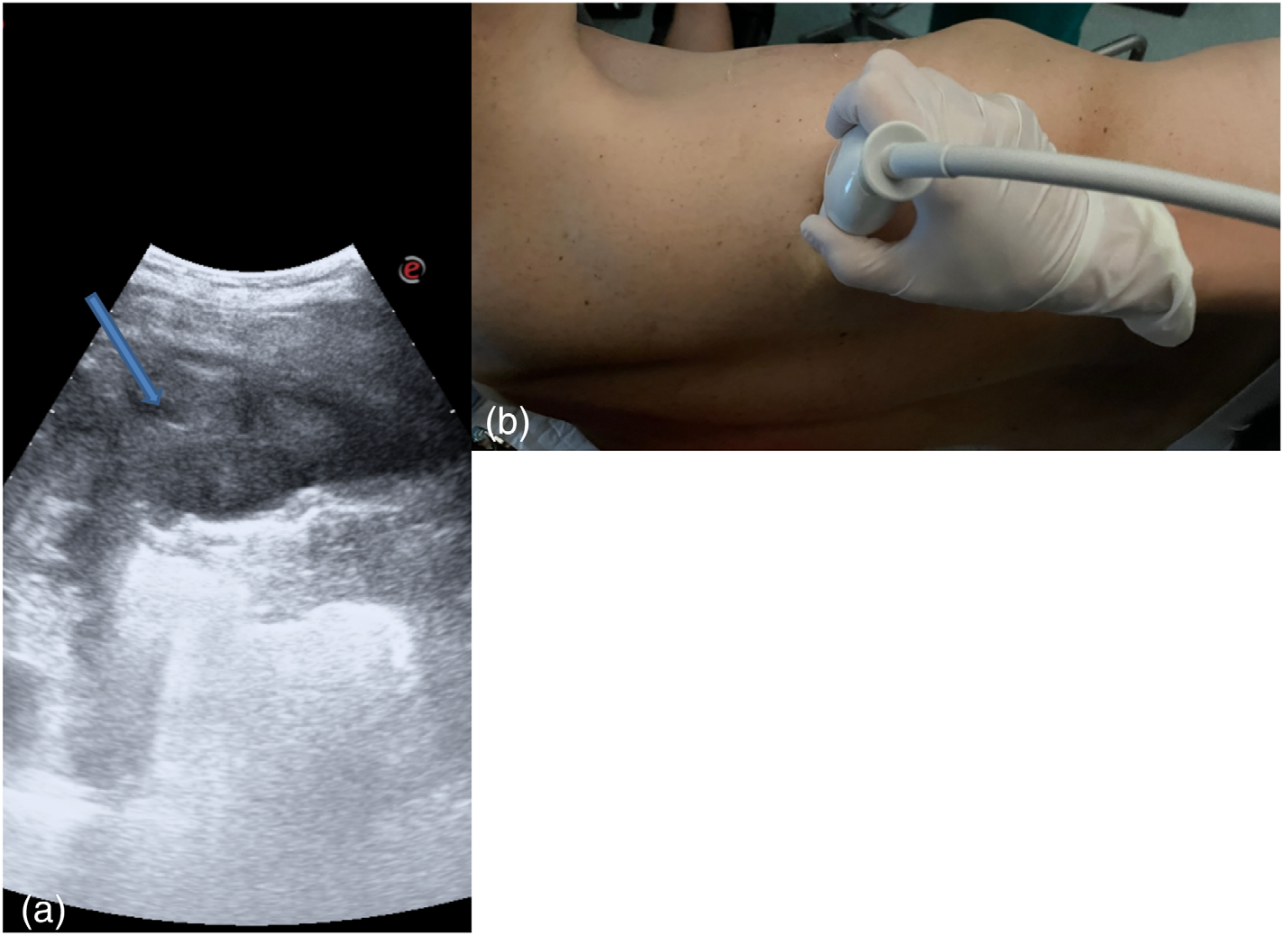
(a) Pleural thickenings were located with transverse or longitudinal scans, moving the probe along or across the intercostal spaces, avoiding interference by bony ribs. (b) Ultrasound information was obtained by tilting and moving the transducer along the intercostal spaces. Within each space two types of probe: convex and linear probes were used.
